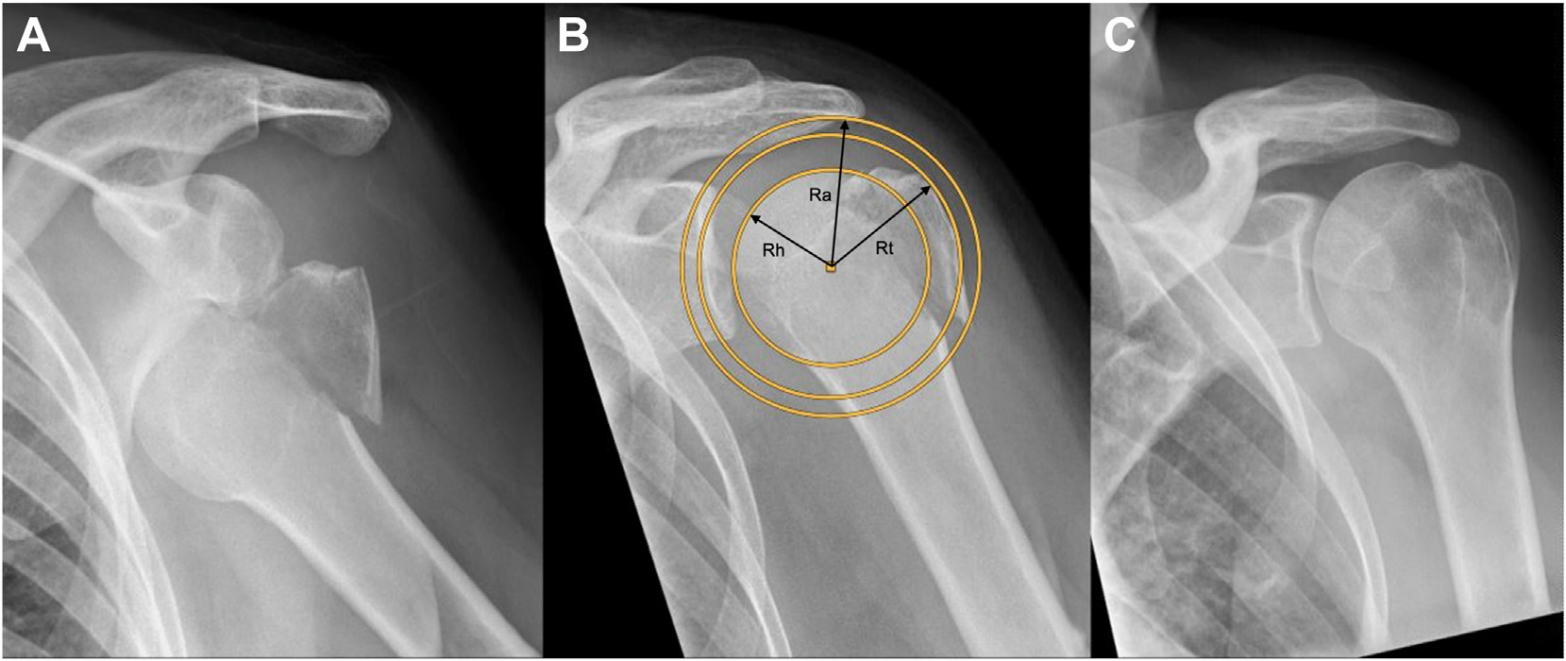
ap radiographs of the glenohumeral joint of two patients showing how the greater tuberosity avulsion fragment after ASD reduces into a close to an anatomic position after closed reduction. From the *Left* to the *Right*: (A) radiograph after ASD, (B) check-up after closed reduction, (C) last follow-up. After closed reduction: GTI: 1.32, II: 1.07. At final follow-up GTI:1.15, II: 0.49. Formula: GTI=Rt/Rh; II=(Rt-Rh)/(Ra-Rh). *ASD*, anterior shoulder dislocation; *GTI*, greater tuberosity index; *II*, impingement index; *Rh*, Radius of a circle tangent to humeral articular surface; *Rt*, Radius of a circle tangent to the greater tuberosity; *Ra*, Radius of a circle tangent to the undersurface of the acromion.
